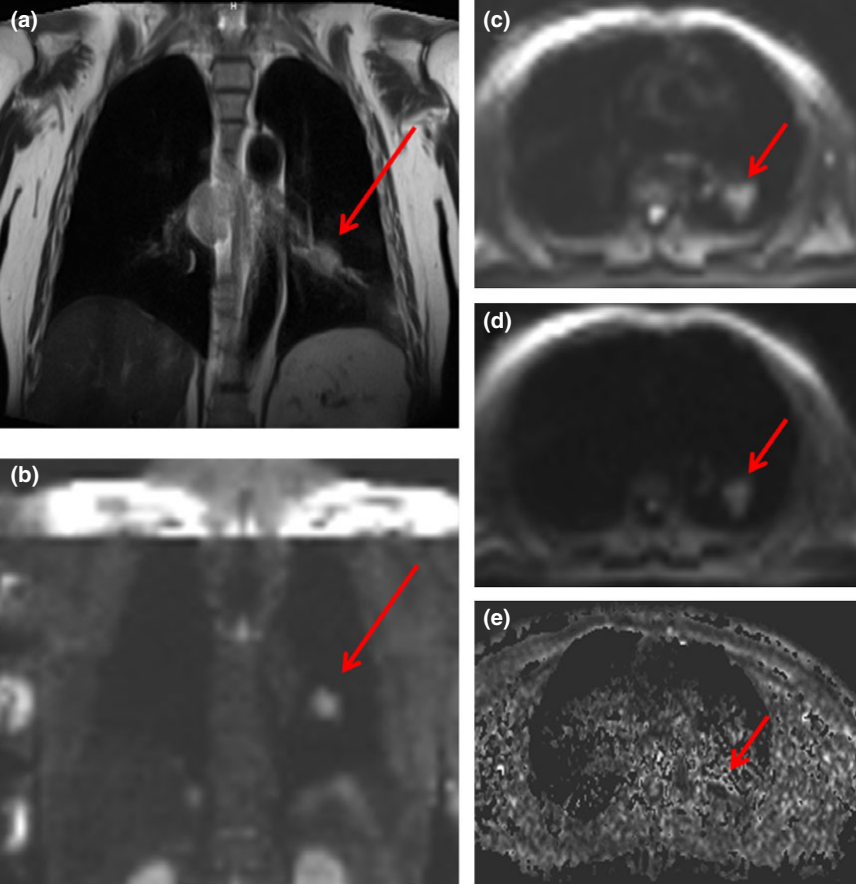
MR scans of a 54-year-old patient with adenocarcinoma in the left lung. During initial examination on coronal T2-weighted images, (a) primary tumour (red arrow) is seen as being isointense to soft tissue. Correspondent coronal DW image displays tumour as a hyperintense zone (b). On the transaxial images, the SI (signal intensity) of the primary tumour (on *b*-value of 50 and 1000 s mm^−2^) is seen to stay relatively high and only decrease slightly together with lesion diameter if *b*-value is elevated (c and d). On ADC (e) map, the primary lesion shows low SI, corresponding to the malignant nature of the tumour.
